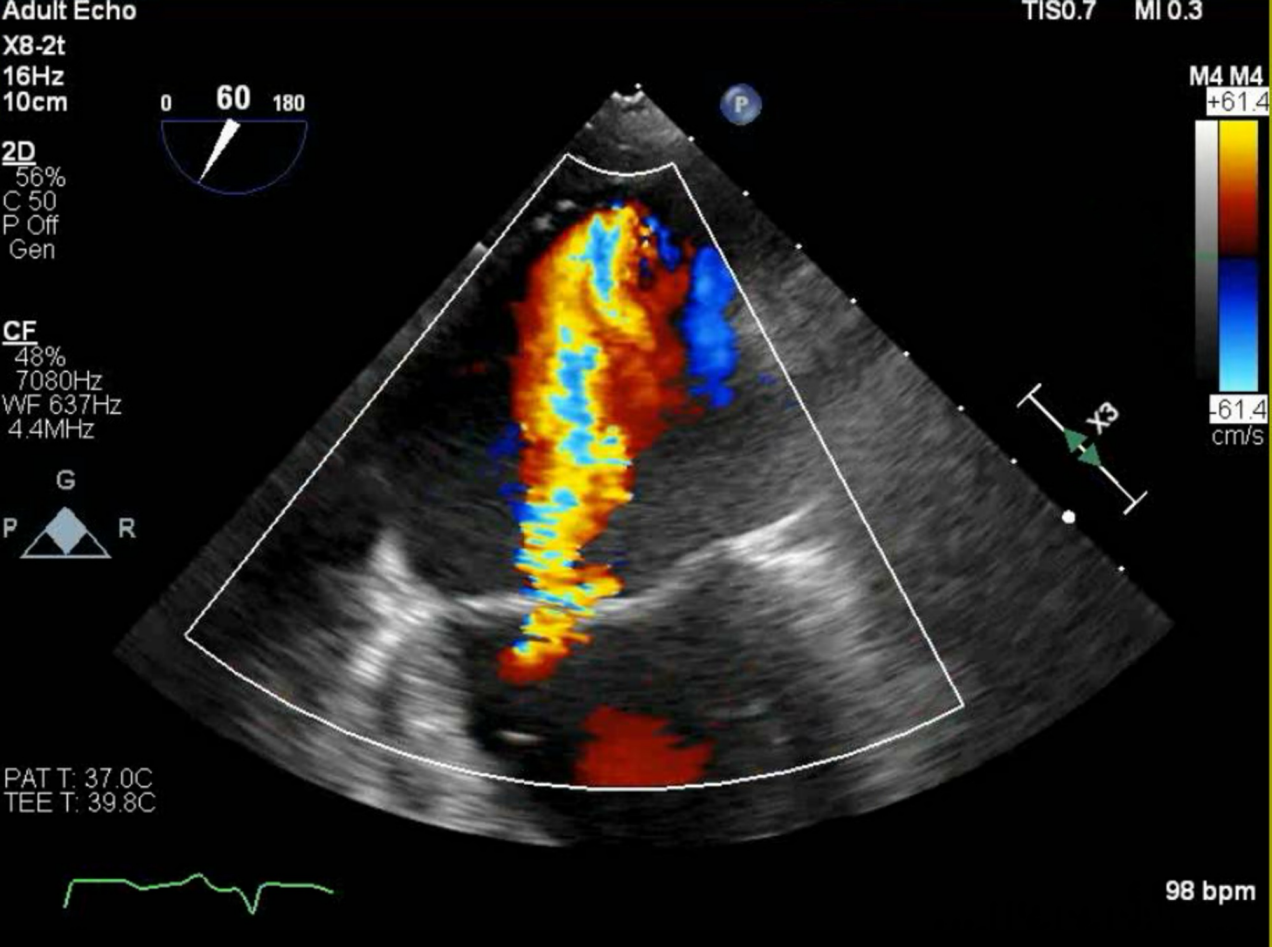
Transesophageal echocardiogram (TEE) This TEE image demonstrates regurgitant flow over the mitral valve.
